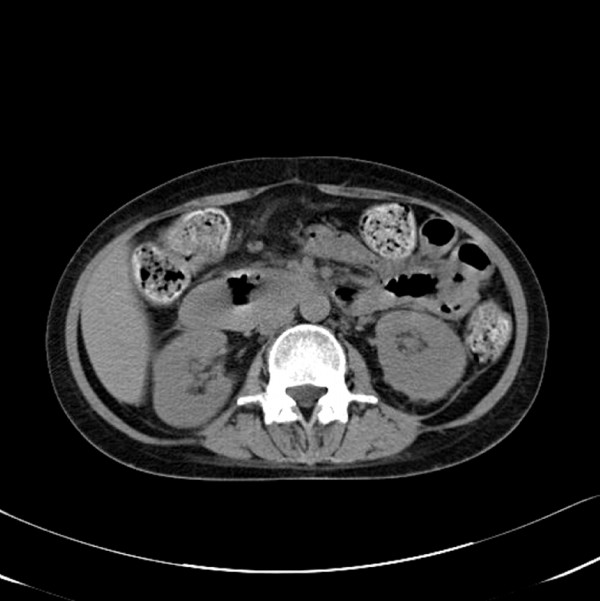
Follow-up CT of the abdomen 12 weeks post-endoscopy demonstrates the decreased size of the hematoma.
